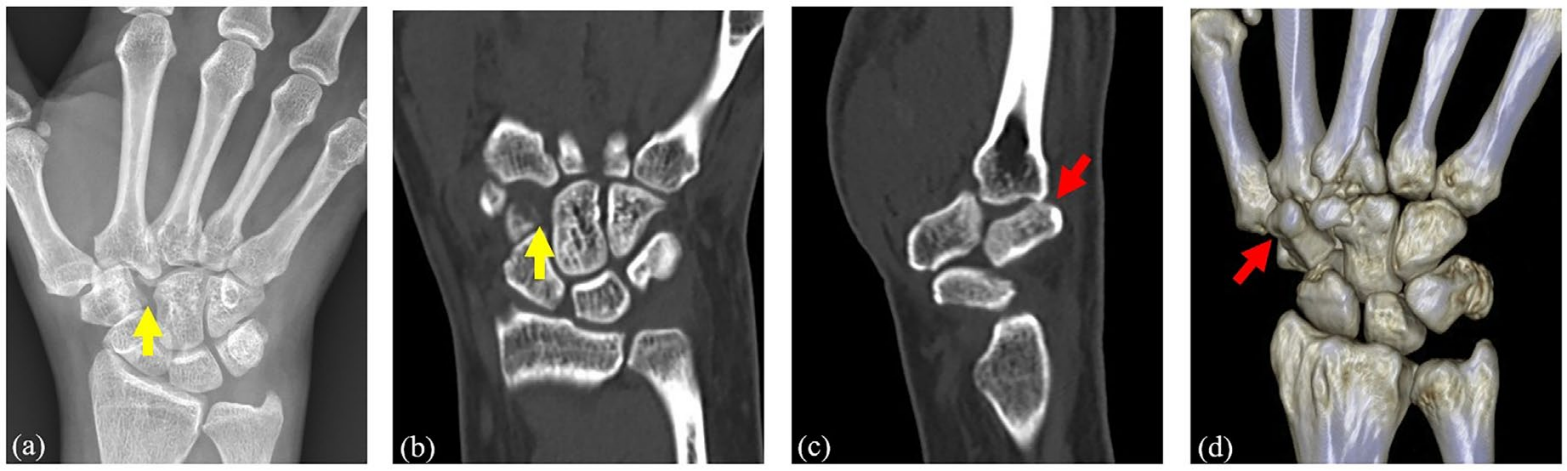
(a, b) Radiographs and CT images show a “missing carpal sign” (yellow arrow). (c, d) CT images show a trapezoid dislocation out of the carpometacarpal joint (red arrow).
